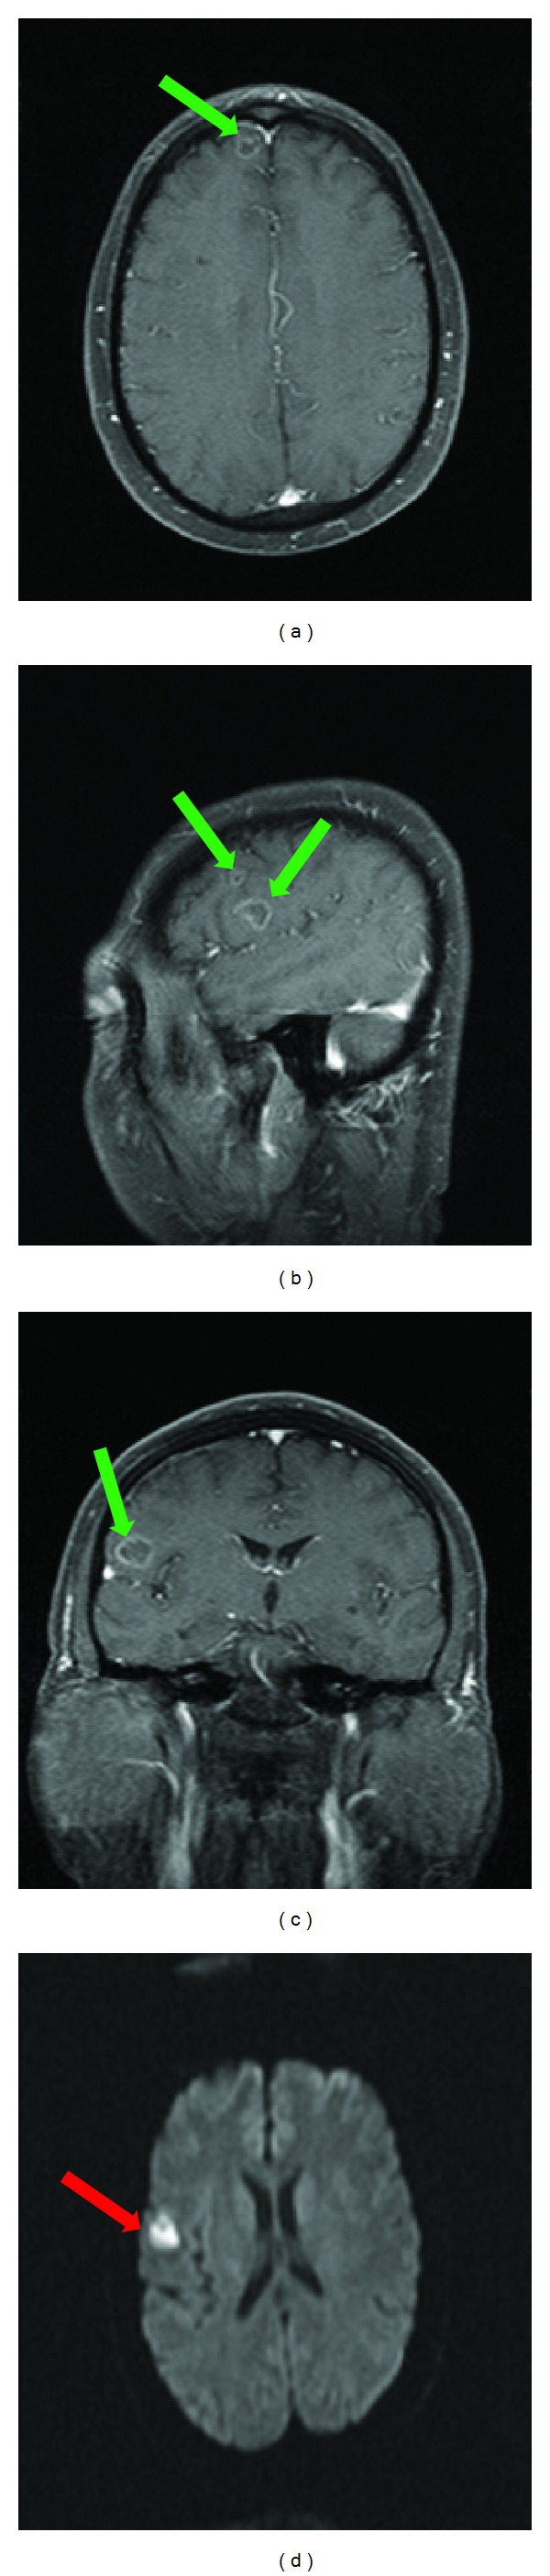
Axial (a), sagittal (b), and coronal (c) T1-weighted fat suppressed images following administration of 15 mL of intravenous gadolinium demonstrates numerous ring-enhancing lesions (green arrows). (d) There is restricted diffusion of the lesions on DWI (red arrow).
